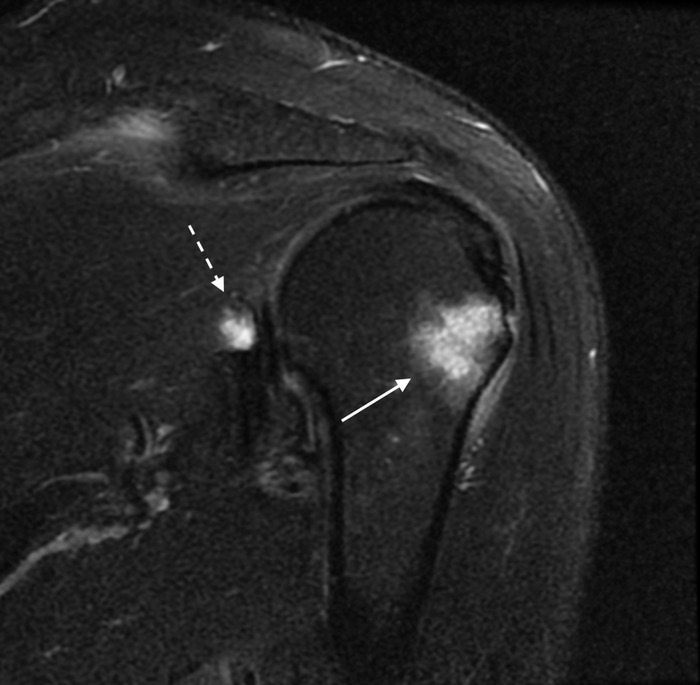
Coronal T2-weighted magnetic resonance imaging of the left shoulder with fat saturation shows focal osseous edema within the humerus at the level of the infraspinatus muscle insertion on the greater tubercle (arrow). A complex paralabral cyst can also be partially seen near the posterosuperior glenoid (dashed arrow).
